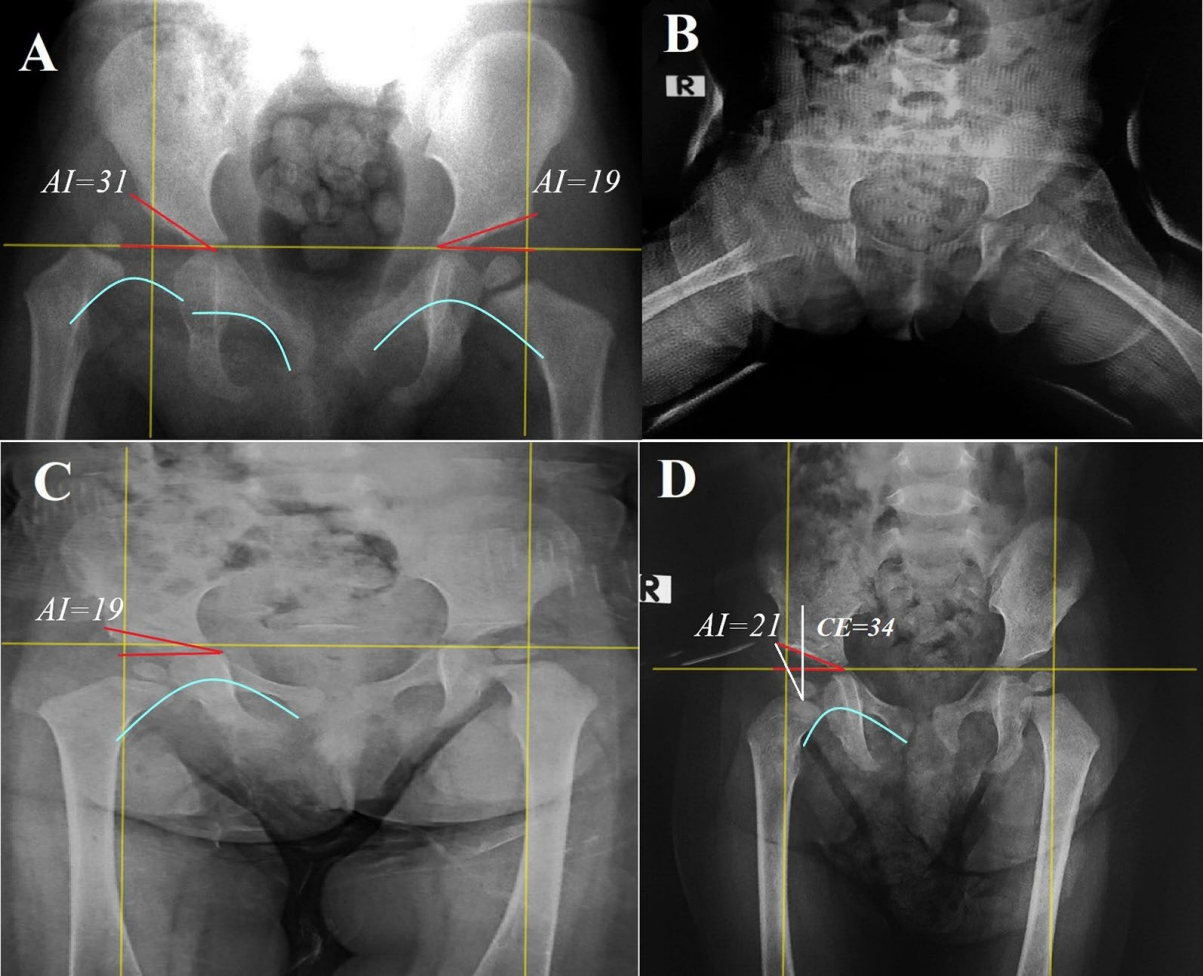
Female child, 20 months, presented with right DDH, Tönnis grade III. A preoperative. B Immediate postoperative. C After 6 months of follow-up, D after 18 months follow up, the radiographic result was Severin’s grade Ι
